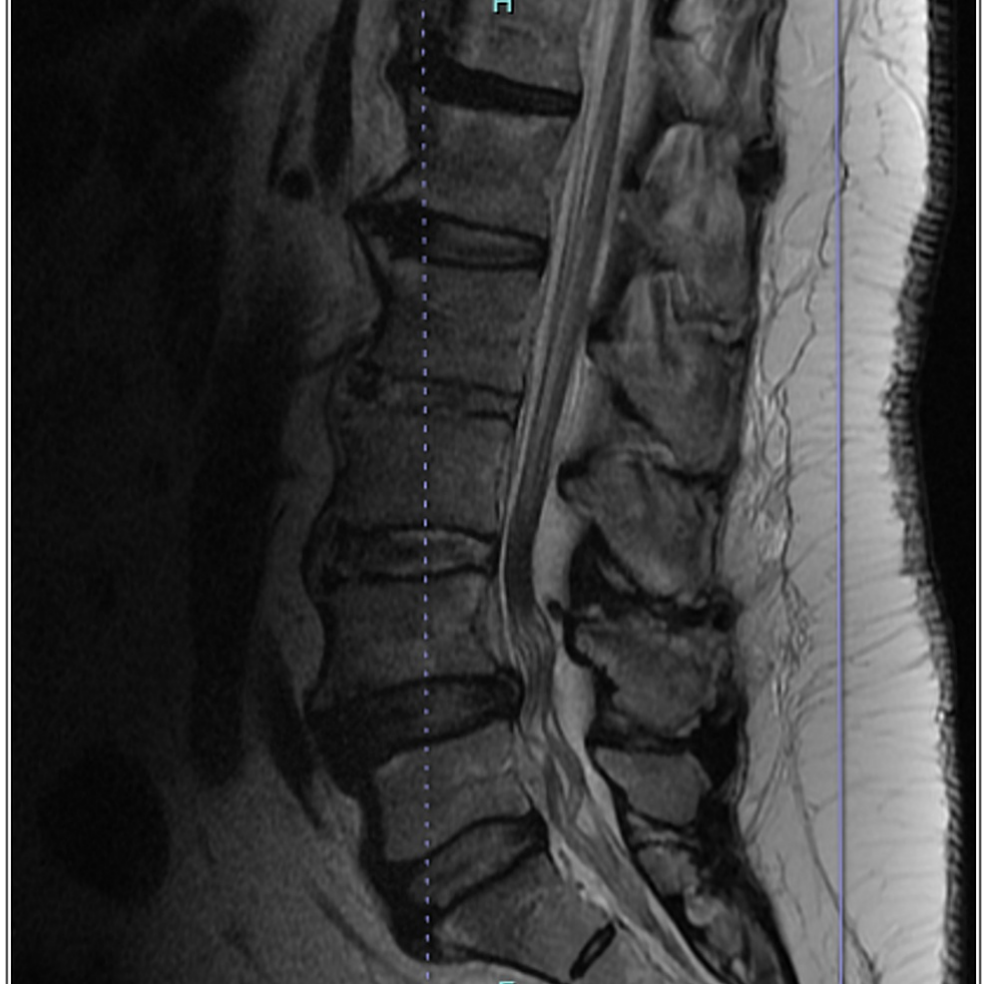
MRI lumbar spine w/wo contrast - shows normal conus medullaris. No unusual contrast enhancement through the cauda equina or proximal nerve roots to suggest neuromyelitis. Multilevel lumbar foraminal stenosis. Clumping of the nerve roots at L3-L4 is a nonspecific finding. There is no contrast enhancement or focal mass. This may be evidence of scarring from focal arachnoiditis. Degenerative disc disease throughout the upper lumbar spine consists primarily of anterior osteophyte formation. No disc herniation.
